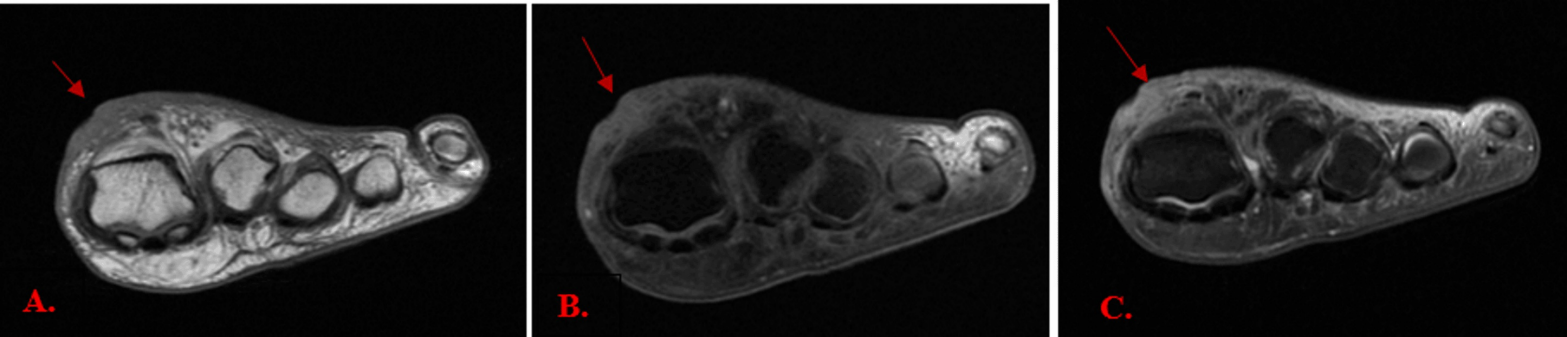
MRI scan images of the left foot in T1, T1FS and PD sequences MRI images of the left foot in the axial view in T1-weighted image (A), in T1-weighted fat-saturated (T1FS) image (B), and in proton density (PD)-weighted image (C), showing a skin ulcer at the dorsum of the great toe with surrounding reactive subcutaneous inflammation (red arrows). No evidence of osteomyelitis appreciated.
